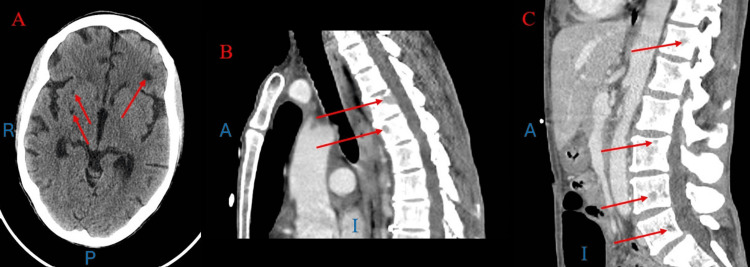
Panel A: Hypodensities in the L frontal and R basal ganglia regions from CT brain without contrast. Panels B and C: Numerous lytic lesions concerning for disseminated infection vs metastatic disease. Panel B at the levels of T4-T5 and Panel C at the levels of T12, L3-L5 from CT chest, abdomen, and pelvis with contrast. Blue letters represent the orientation of the image; R: Right; P: Posterior; A: Anterior; I: Inferior
